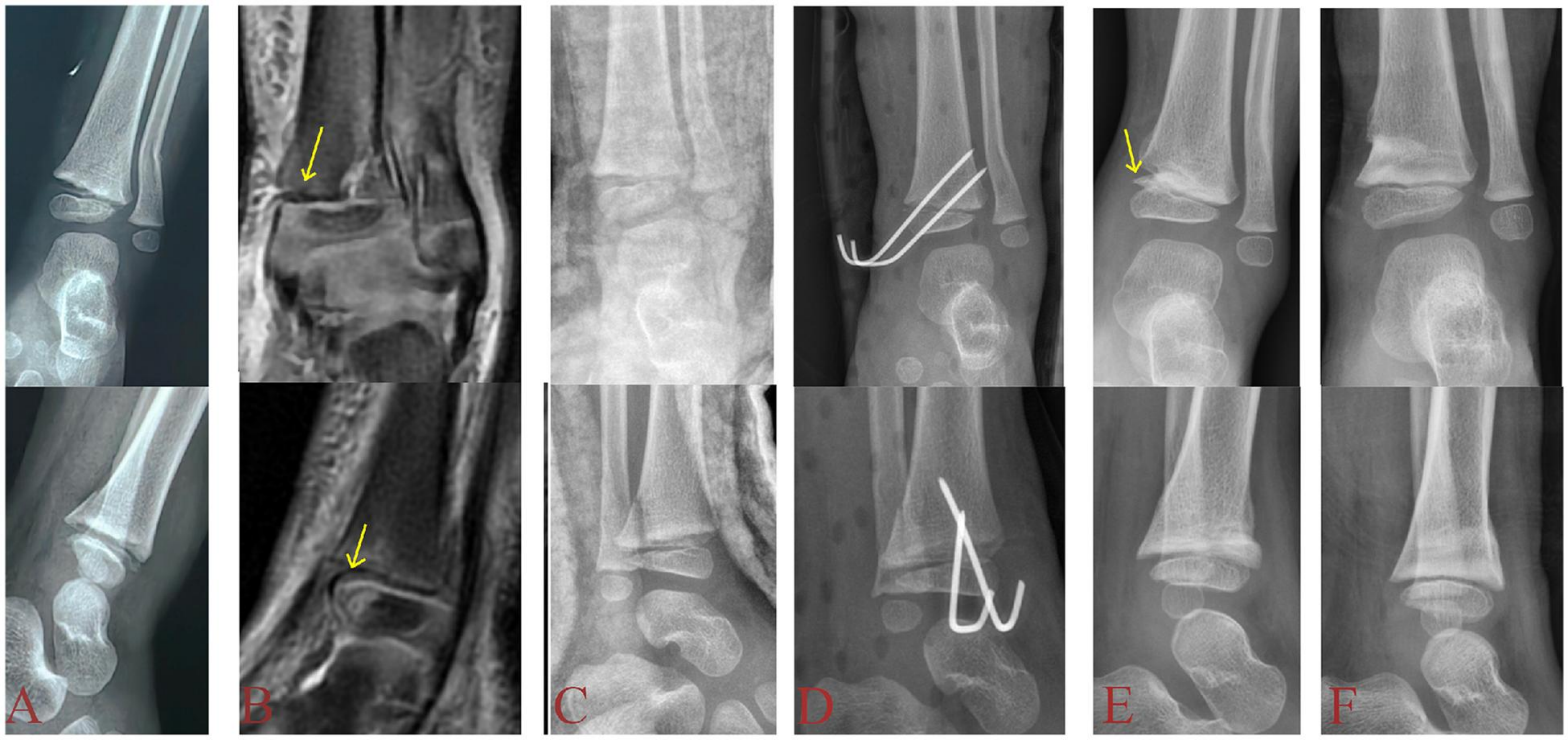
A 2-year-old boy presented with an open distal tibial S-H type II fracture (PEER injury). (A): Initial radiograph shows widening of the anteromedial physeal gap of the distal tibia. (B): MRI reveals periosteal entrapment within the anteromedial quadrant of the distal tibial physis (yellow arrow). (C): Following closed reduction, repeat radiograph shows persistent widening of the anteromedial physeal gap. (D): CR-PF was performed under general anesthesia, achieving satisfactory postoperative joint alignment. (E): Two-month follow-up radiograph shows the “periosteal entrapment sign”—a horizontally oriented bony protrusion with density comparable to normal bone in the anteromedial distal tibia, distinct from the circumferential callus formation seen in subperiosteal ossification (yellow arrow). (F): Six-month follow-up demonstrates excellent ankle function without angular deformity.
